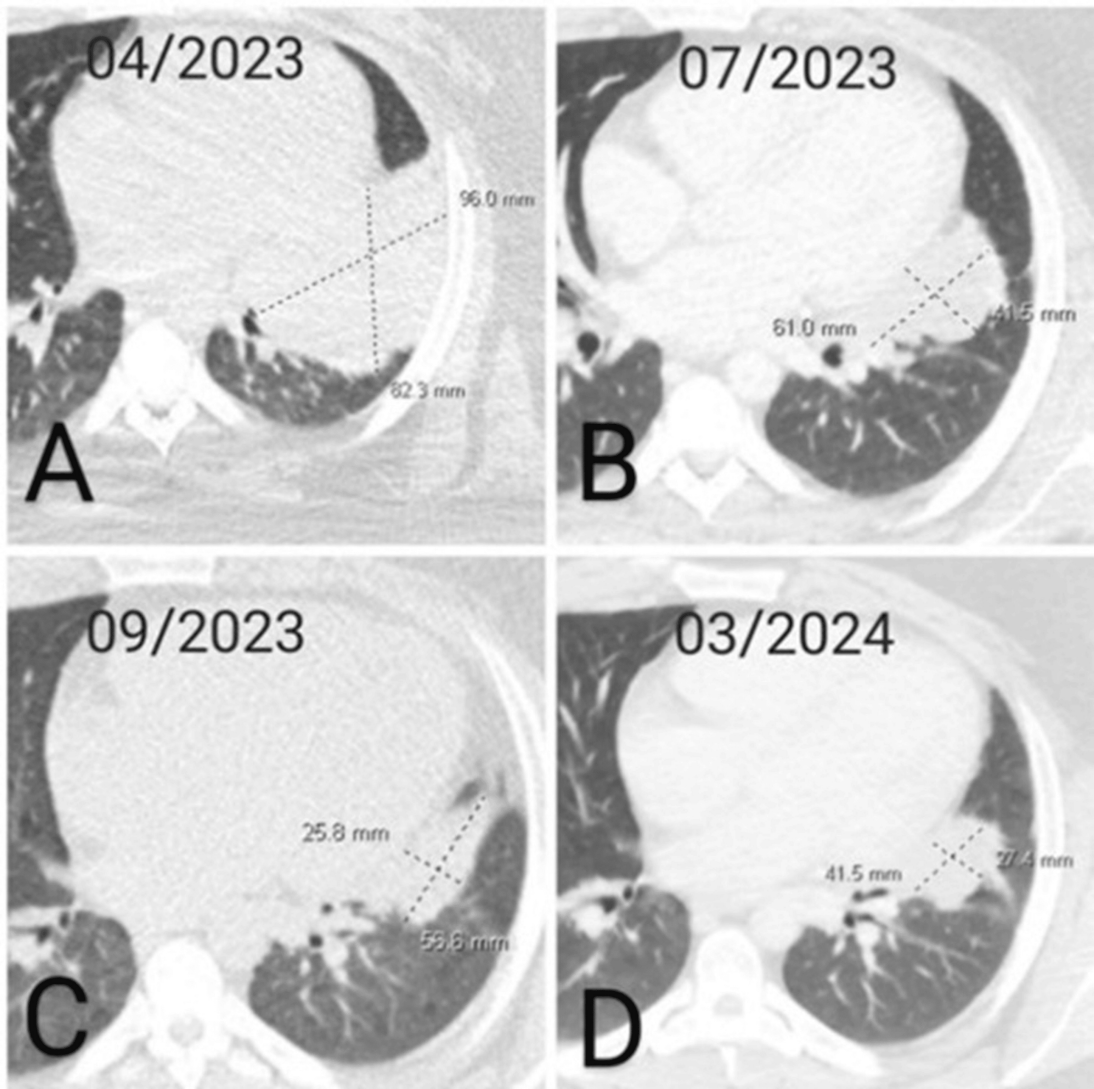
CT chest evolution from April 2023 to March 2024
